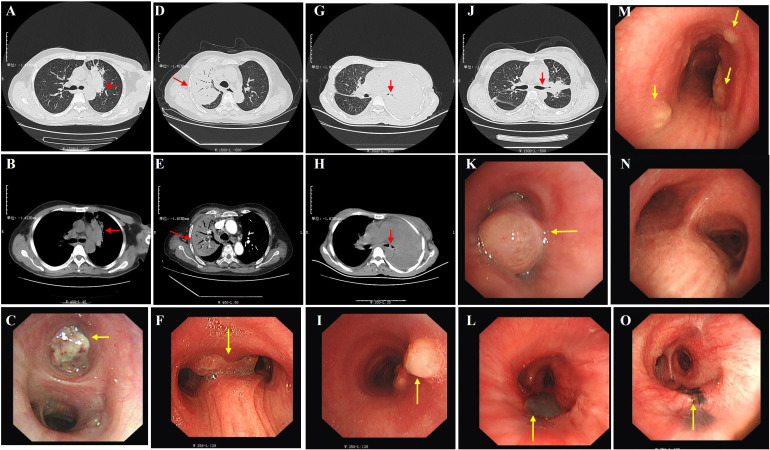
(A, B) Chest CT of Patient 1 revealed irregular mass-like consolidation at the left hilum with narrowing of the left main bronchus. (C) Bronchoscopy in Patient 1 revealed a polypoid mass completely occluding the left upper-lobe orifice, with a rough surface that bled on contact. (D, E) Chest CT of Patient 19 revealed left-sided thoracic cage collapse, extensive consolidation, and complete luminal occlusion, with a nodular lesion visible in the left main bronchus (arrow); (F) bronchoscopy demonstrated a smooth-surfaced neoplasm obstructing the same bronchus. (G) Follow-up chest CT after anti-NTM therapy showed left upper lobe re-expansion, a reduction in pulmonary lesions, resolution of the nodule, and a patent airway. (H) Bronchoscopy of Patient 2 revealed multiple, variable-sized nodular lesions diffusely distributed along the distal tracheal wall; (I) follow-up bronchoscopy after anti-NTM therapy revealed complete regression of these previously noted tracheal masses. (J) Bronchoscopy of Patient 6 revealed two discrete polypoid masses within the mid-tracheal lumen. (K) Bronchoscopy of Patient 16 revealed a widened carina with an irregular mass extending into both main-stem bronchi, causing bilateral proximal bronchial stenosis. (L) Bronchoscopy of Patient 18 revealed stenosis of the right middle-lobe orifice, with associated mucosal swelling, thickening, and surface ulceration. (M) Diffuse variably-sized nodular lesions in the lower trachea (Patient 2). (N) Complete resolution of lower tracheal lesions after 2-month anti-NTM therapy. (O) Resolution of the brownish lesion at the membranous portion of the right intermediate bronchus (Patient 18) following anti-NTM therapy.
